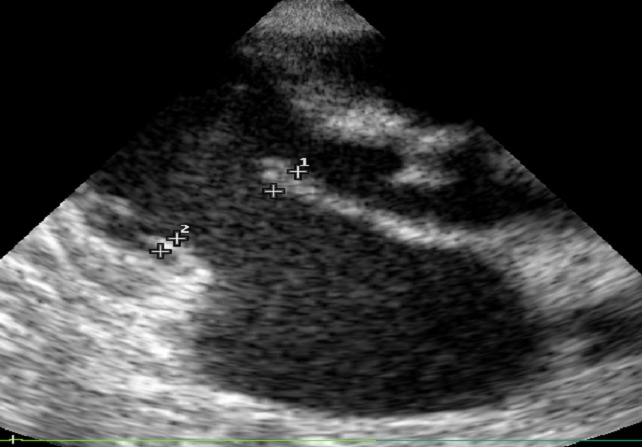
Parasternal long axis echocardiographic view showing the measurement of the anterior (AML) and posterior (PML) mitral leaflets
